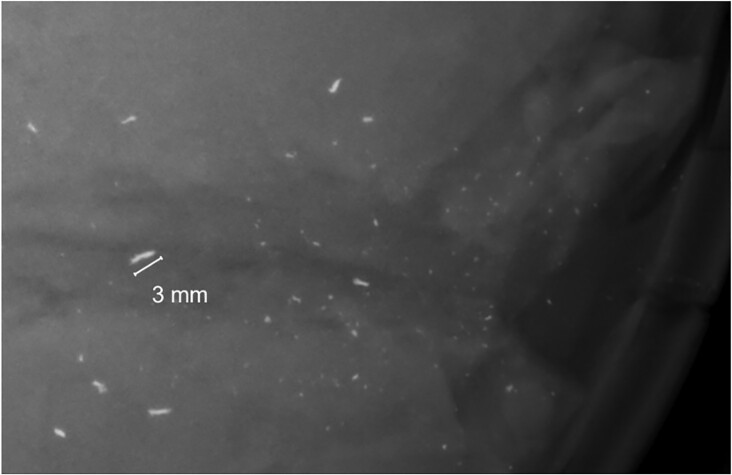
Remnants in a radiograph were typically submillimeter in size. Largest streaky fragments in experiment 3 were around 3 mm in length.
